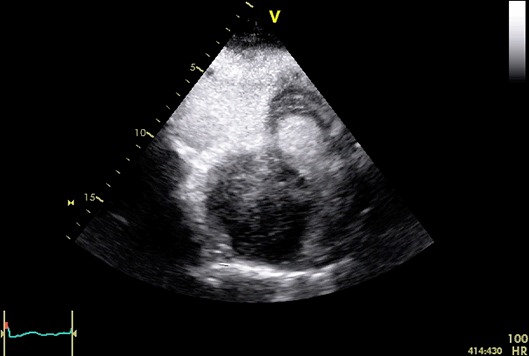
Transthoracic echocardiography, off-axis view of the right atrium, acquired at the end of contrast infusion, showing perfusion of the mass
